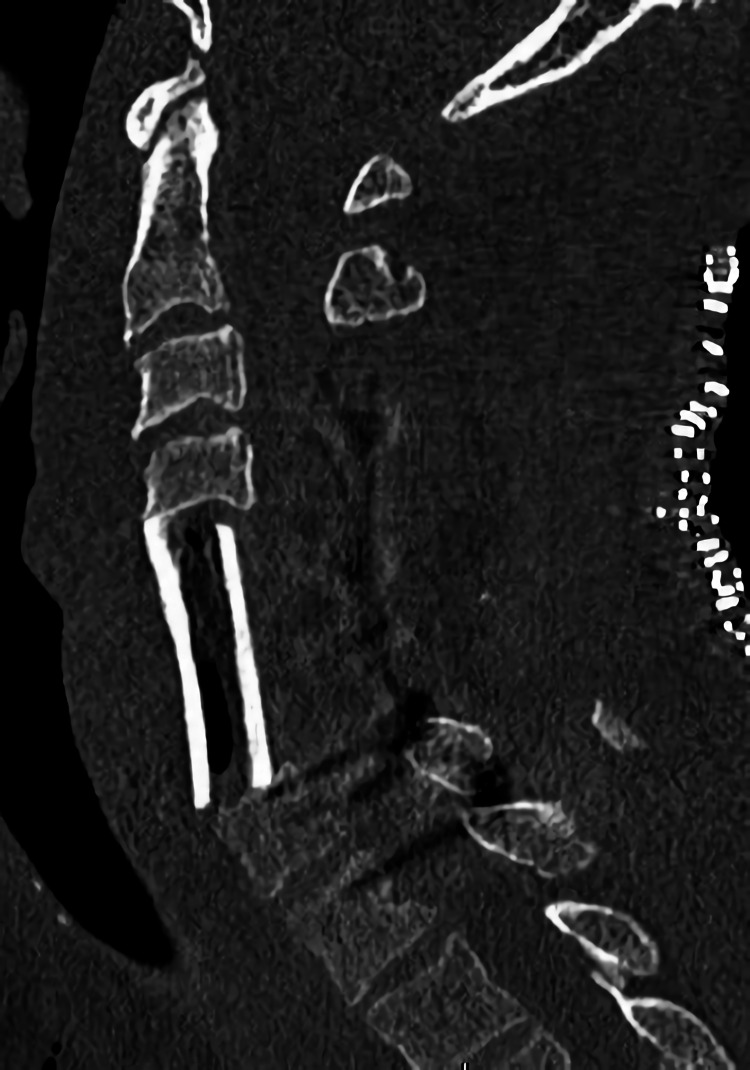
Cervical plain CT scan after the third surgery Sagittal view of the cervical CT scan after the third surgery of anterior corpectomy and fusion with an autologous long bone graft.
